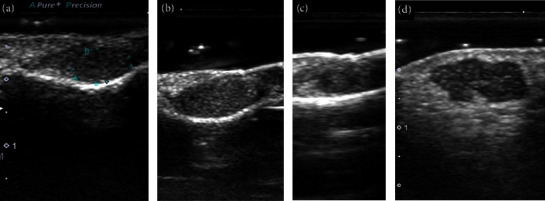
Representative ultrasound images of tumour shapes: (a) regular (ellipse), (b) regular (squamous), (c) regular (round), and (d) irregular.
